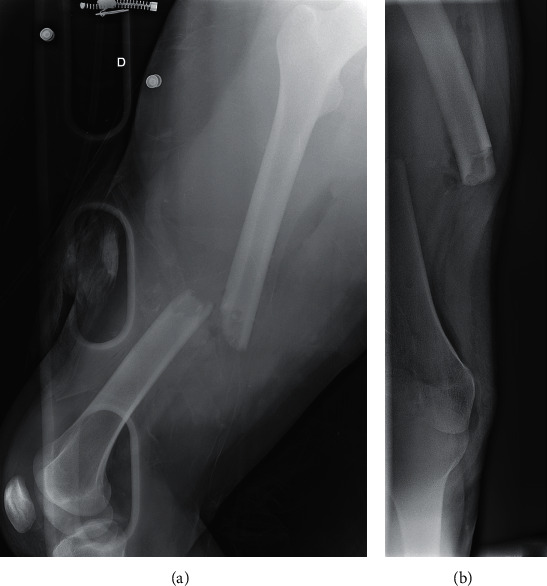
Anteroposterior (a) and lateral (b) X-rays of a Gustilo-Anderson III-A open fracture of the femur in a 16-year-old female patient.
